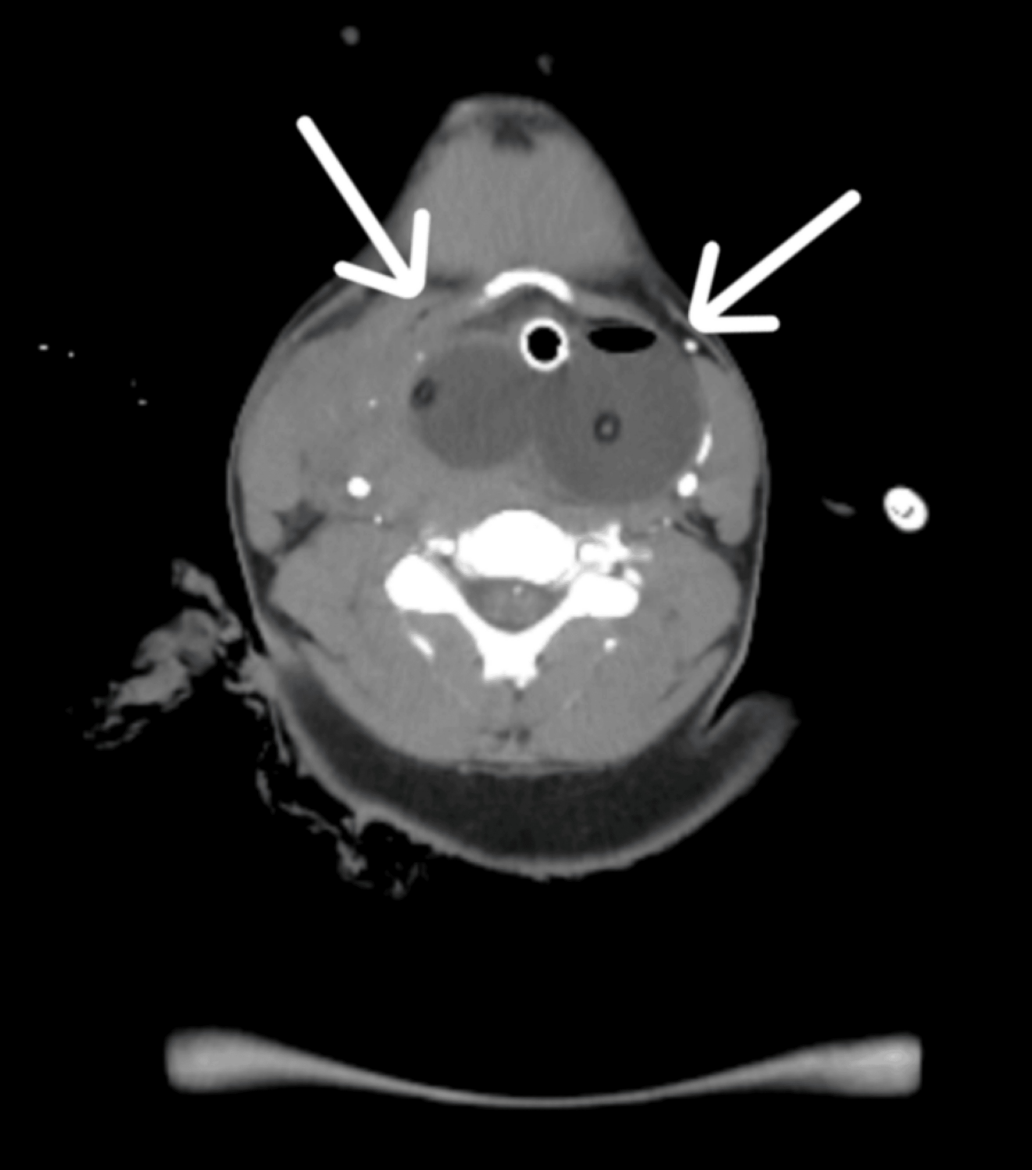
Two pharyngeal Foley balloon catheters in situ at the bleeding site. CT image showing two pharyngeal Foley balloon catheters in situ at the bleeding site (arrows), achieving immediate tamponade and hemostasis. CT, computed tomography
